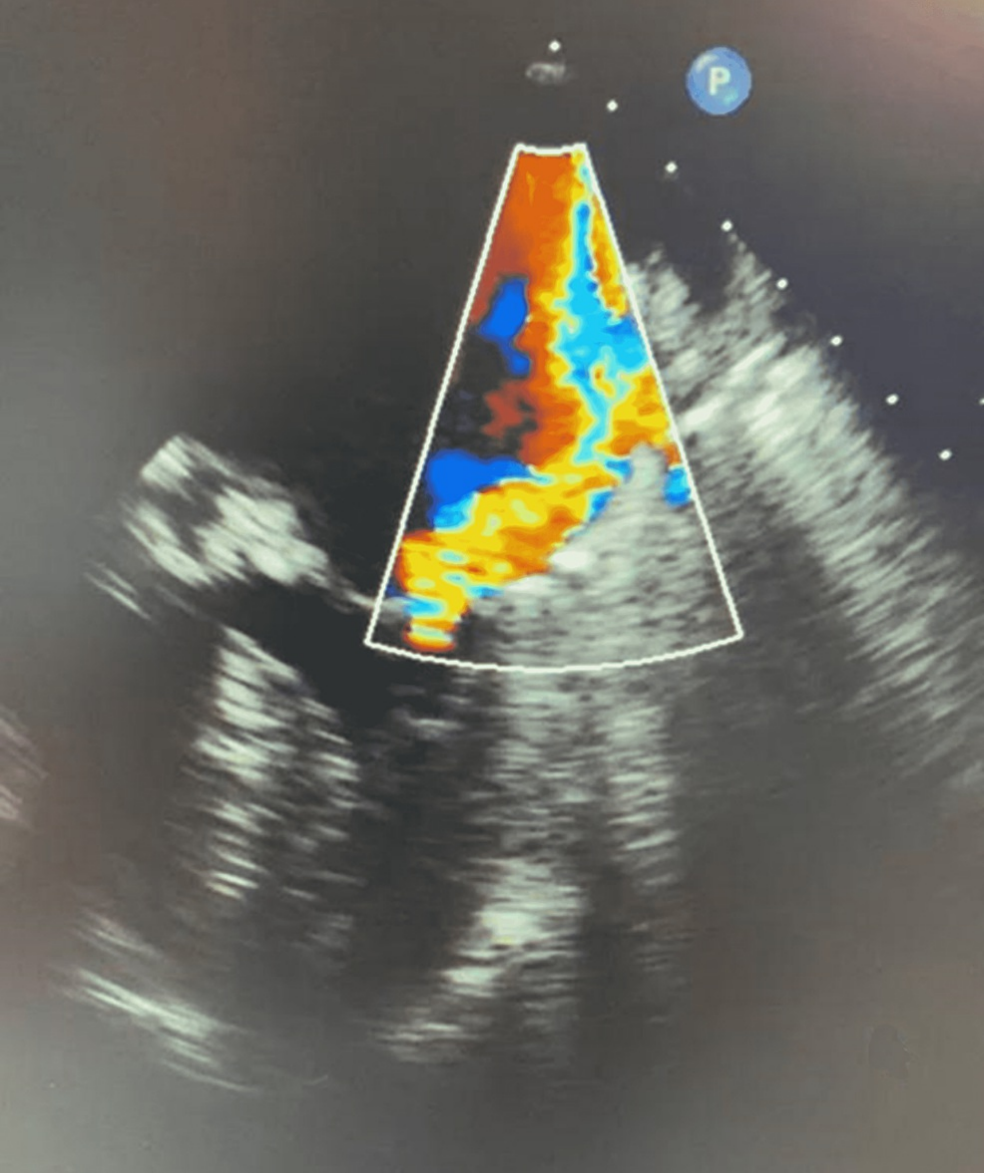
Transoesophageal echocardiogram demonstrating posteriorly directed eccentric jet of severe mitral regurgitation including flow reversal into the left lower pulmonary vein.
